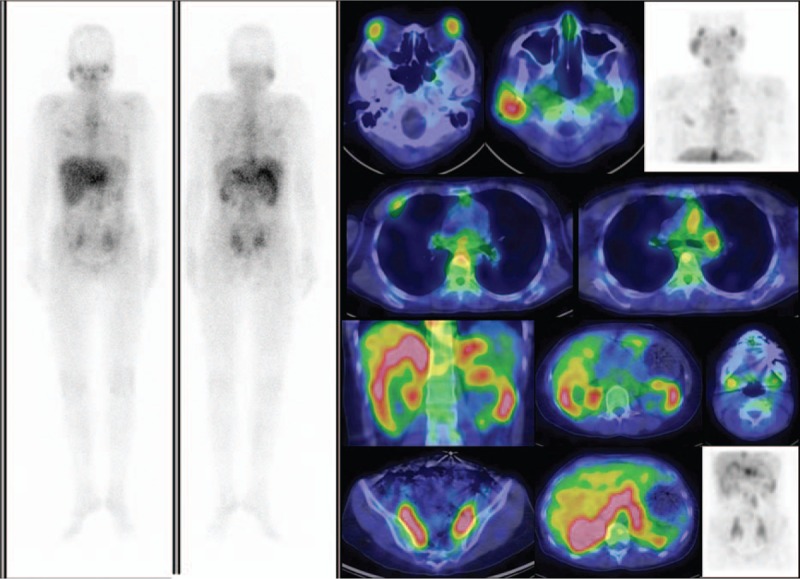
Pre-therapy gallium scan with SPECT/CT showed increased uptake in lacrimal, right parotid, and bilateral submandibular glands, and mediastinal, prevertebral, paraaortic and bilateral pelvic (including internal iliac chain) lymph nodes. There was also uptake in the 3rd and left 6th ribs, and renal and perirenal areas.
